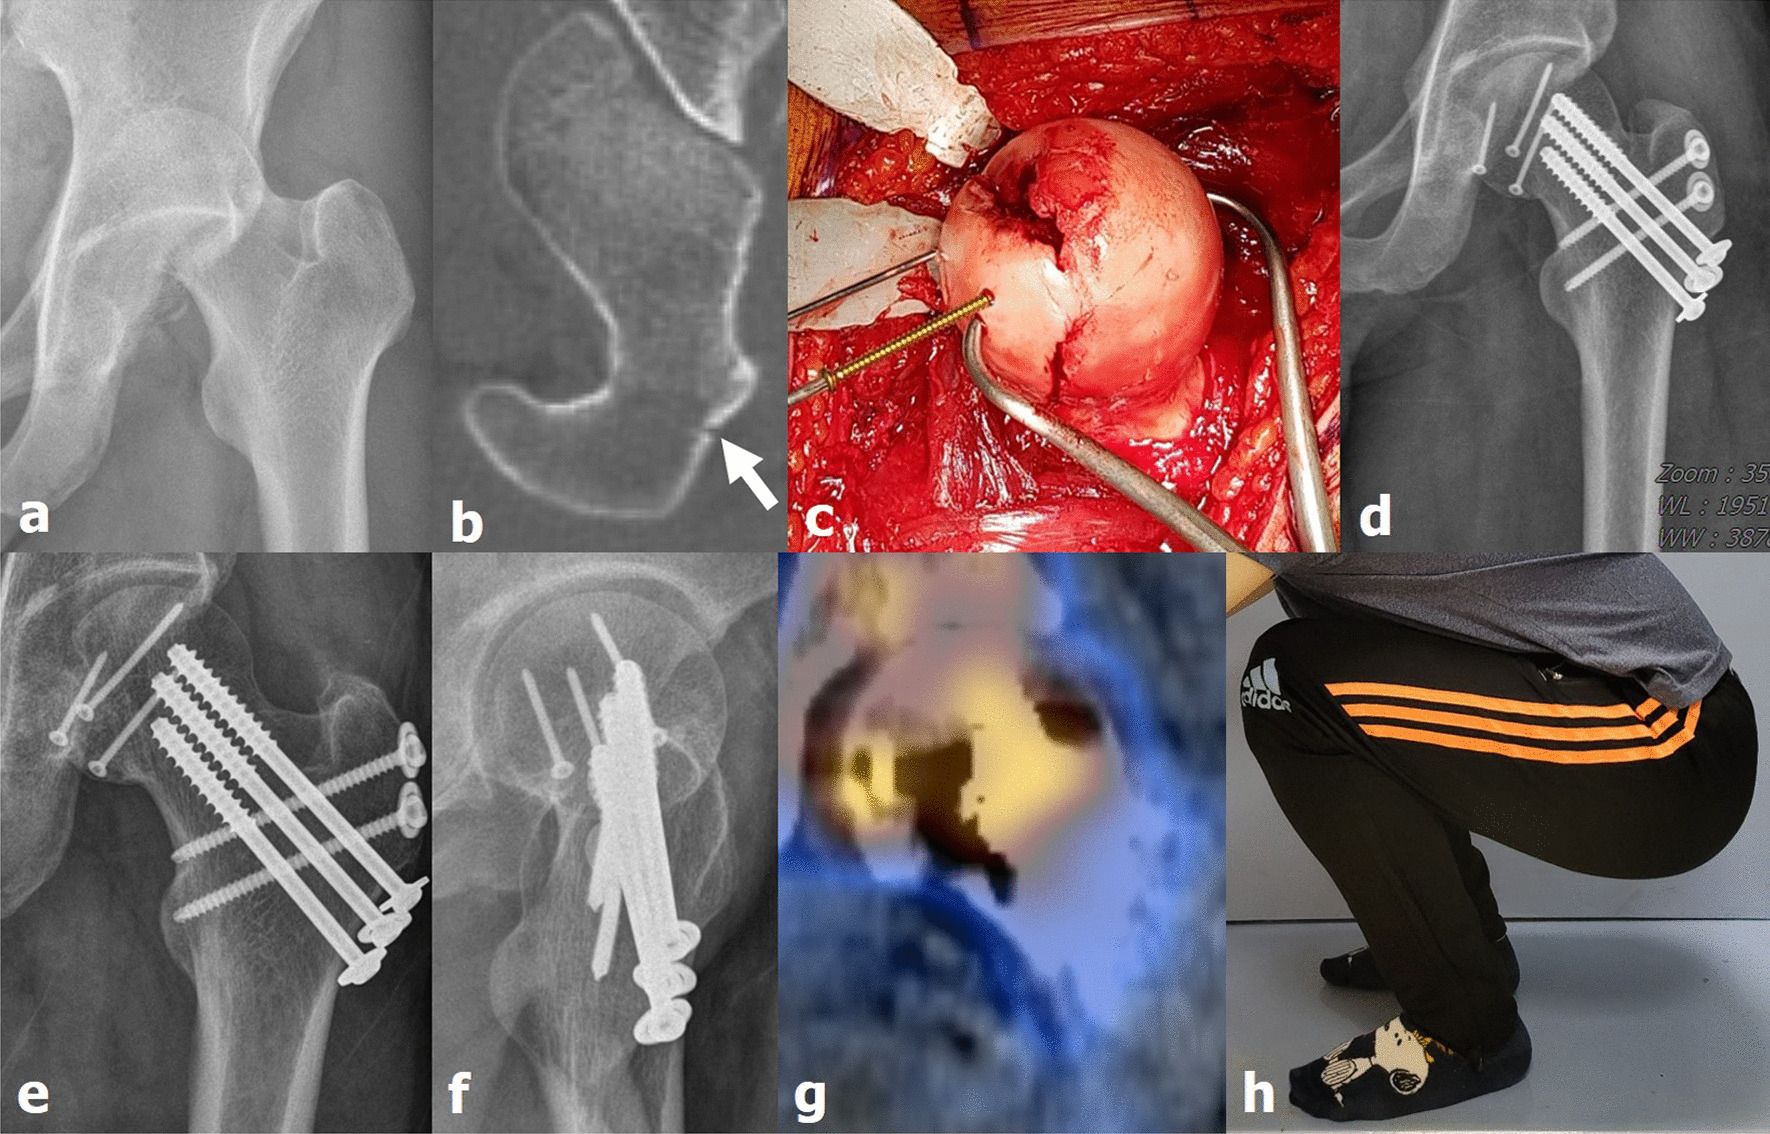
(a, b) Pipkin type III femoral head fracture and dislocation and femoral neck fracture (white arrow) in the left hip joint owing to an in-car traffic accident (case 9, a 31-year-old man). (c) After performing surgical hip dislocation with trochanteric flip osteotomy, the femoral head was exposed and fixed with cortical screws. (d) Femoral neck fracture was fixed using three cannulated screws. (e, f) Bone union was achieved 4 months after surgery, and (g, h) blood supply to the femoral head was found to be well maintained on single-photon emission computed tomography/computed tomography. The patient fully recovered range of motion
